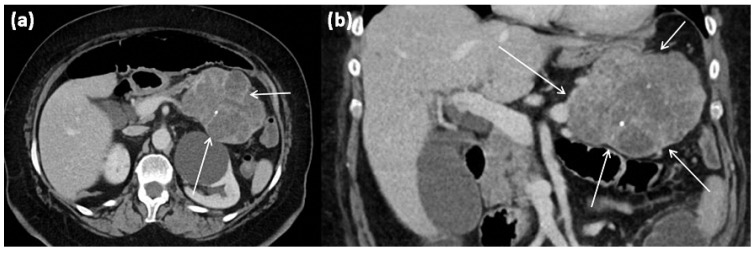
CT of a 63-year-old female patient (portal venous phase) in axial (a) and coronal (b) view. CT shows a large polylobulated tumor of the pancreas tail with partly cystic parts (arrows). In addition, single punctate calcifications and sharp demarcation to surrounding tissue can be seen.
